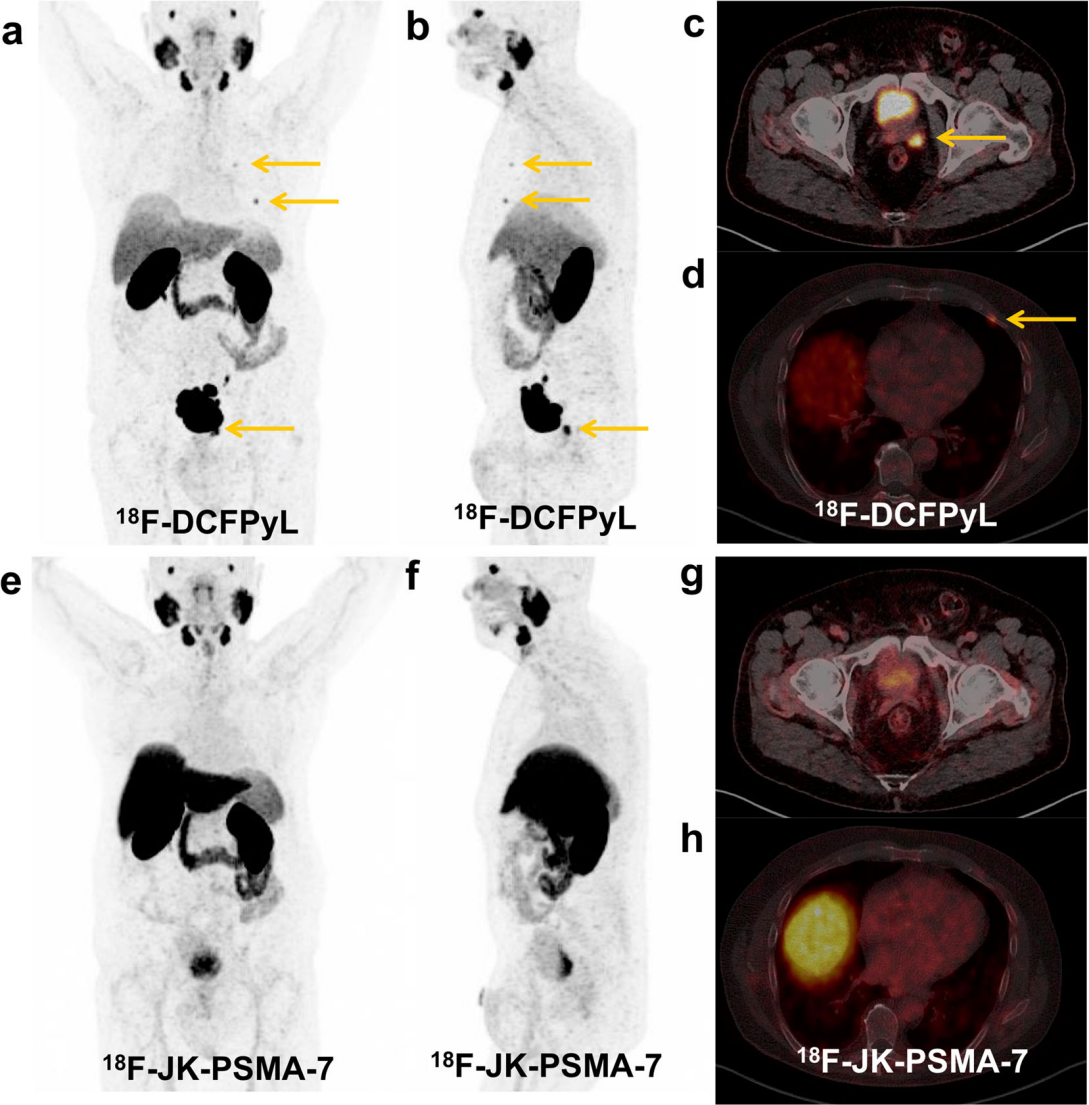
a, b, c, d [^18^F]-DCFPyL PET/CT (top) and e, f, g, h [^18^F]-JK-PSMA-7 PET/CT (bottom). The hormone-sensitive patient received irradiation due to the PSMA-positive relapse in the left seminal vesicle and ADT due to the PSMA-positive skeletal metastases in the 2nd and 4th ribs left. The [^18^F]-JK-PSMA-7 PET scan after 6 months became completely PSMA negative. The PSA level has dropped to 0.012 ng/ml.
